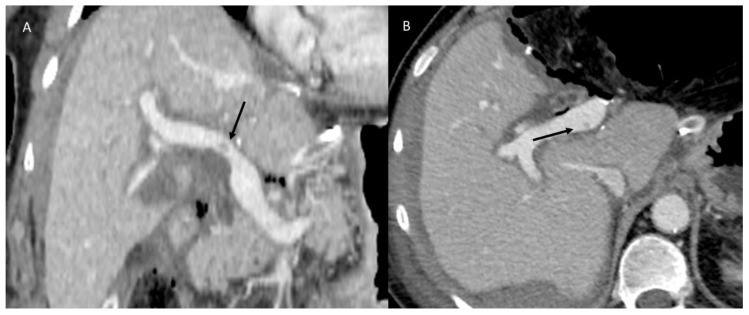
CT portal phase assessment in resected liver metastases patient. The arrow shows (A,B) mild portal thrombosis.
